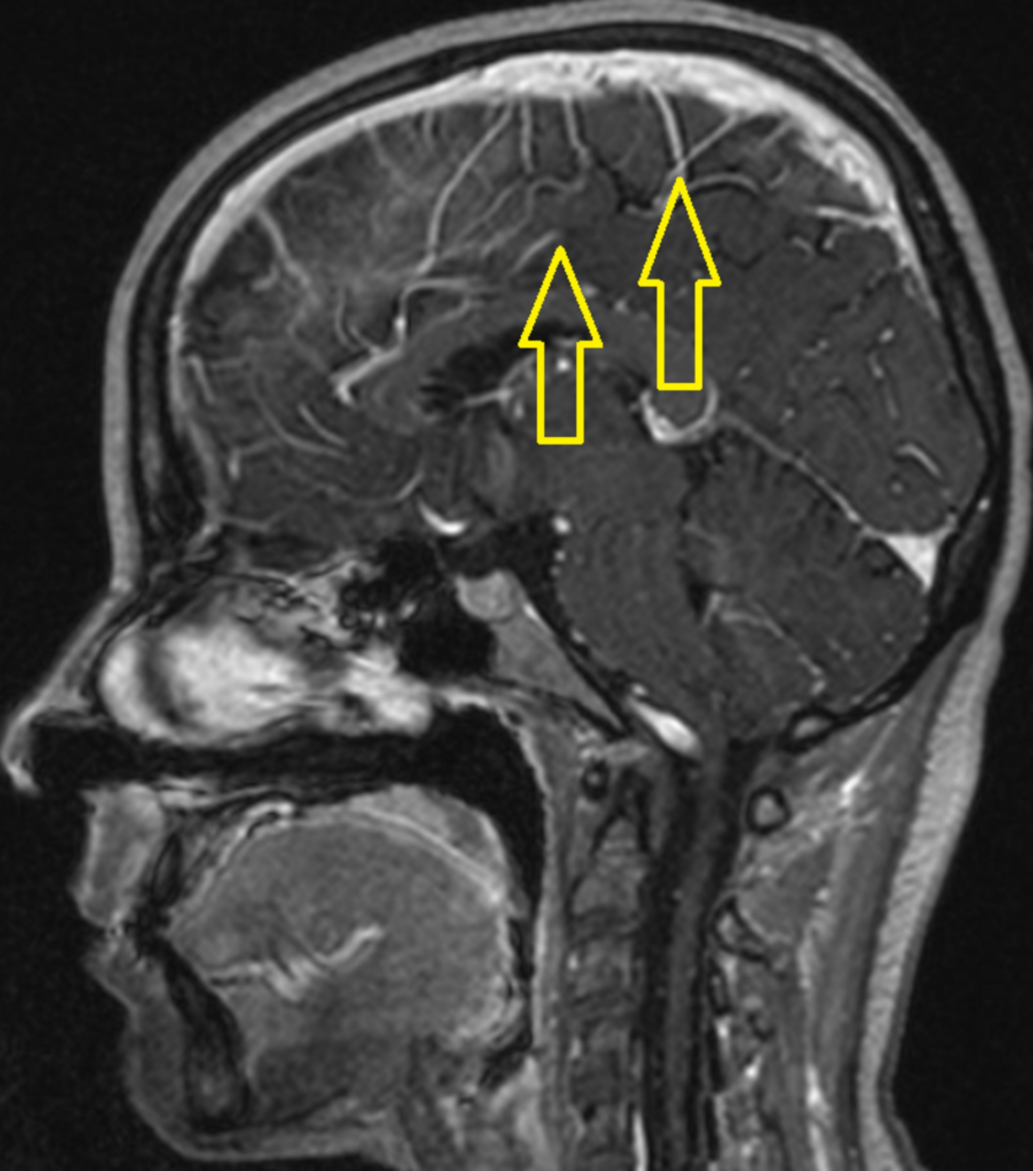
The sagittal post-contrast T1 weighted brain MRI image demonstrates leptomeningeal enhancement (yellow arrows).
